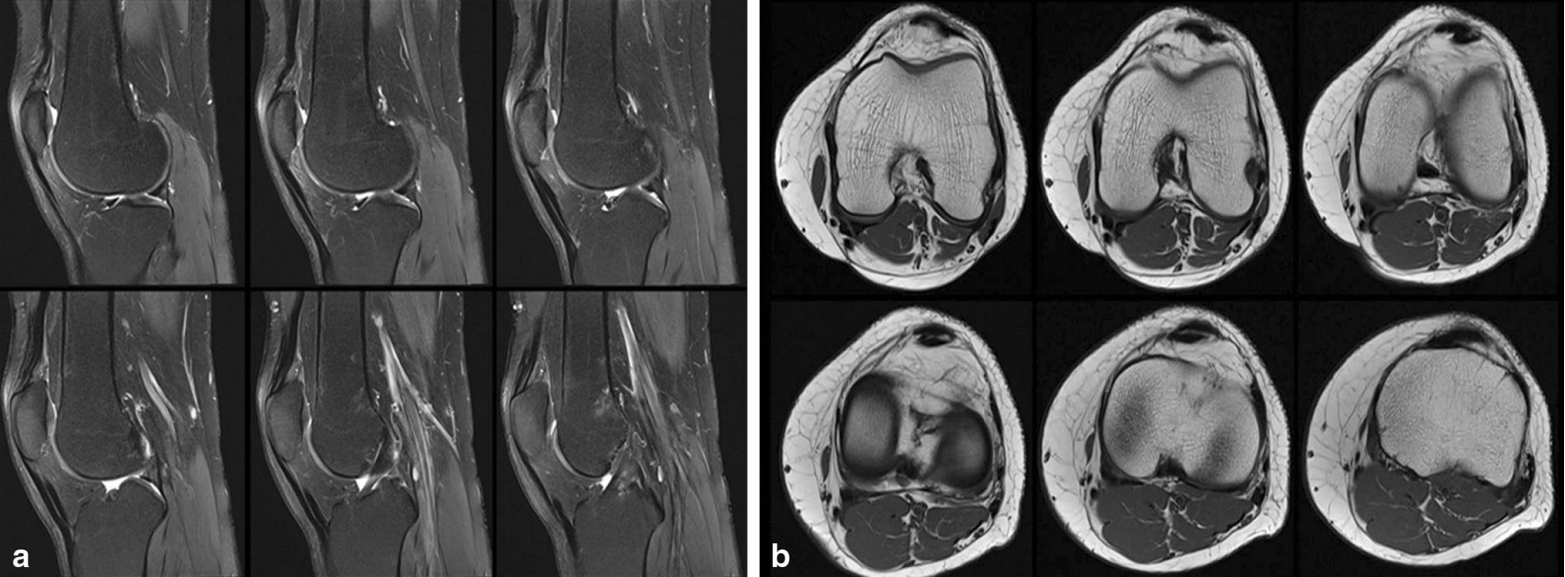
Sagittal intermediate-weighted fat-suppressed images (TE 38 ms; TR 3950 ms; matrix 448 × 448, slice thickness 3 mm) of the right knee in six different slice positions (a). A bifid, low-signal intensity, band-like structure, separated by a thin fat strip along the whole course of the PT can be shown on each image. Axial T1-weighted images (TE 19 ms; TR 626 ms; matrix 448 × 378, slice thickness 3 mm) of the right knee in six different slice positions starting at the height of the femur condyles (*first row*, *first image*) and running distally until the tuberosity of the tibia (*second row*, *third image*), and depicting the two crossing bundles of the patellar tendon (b)
